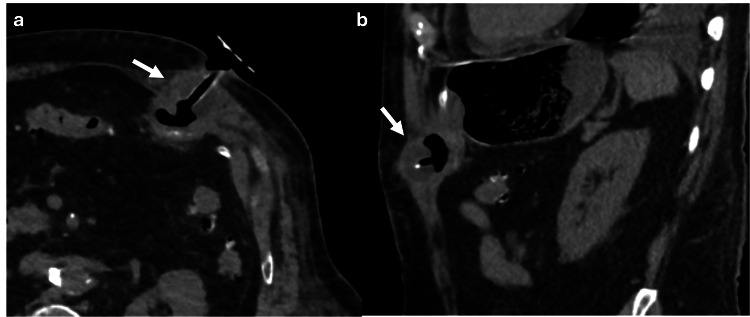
Axial non-enhanced abdominal CT scan showing a buried bumper syndrome (a, b) A 91-year-old male with a history of gastrostomy tube insertion presents with gastrointestinal bleeding and abdominal pain; notice the migration of the gastrostomy balloon and internal bumper into the rectus abdominis muscle (white arrows), leading to entrapment of the gastric mucosa, these findings collectively are suggestive of buried bumper syndrome. The figure was created by the authors.
